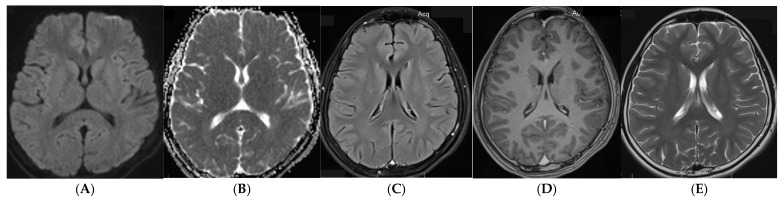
(A–E): Repeat MRI at 6 months of treatment. Diffusion weighted (A), ADC (B), axial FLAIR (C), Axial T1W post Gad (D) and Axial T2W (E) images show a complete absence of lesions previously seen in Figure 1.
